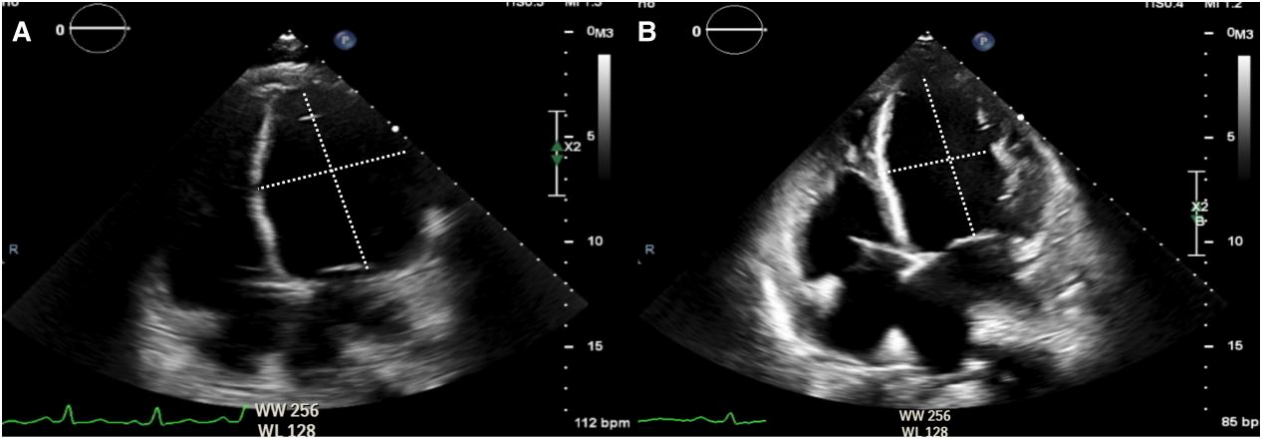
Two-dimensional echocardiography showing the apical 4-chamber view with the baseline echocardiography before treatment (A) and the repeat echocardiography after 10 sessions of octreotide long-acting repeatable (B). The left ventricular end diastolic volume was 6.0 cm and 6.4 cm before and after treatment, respectively (normal value, 3.9-5.3 cm for females).
